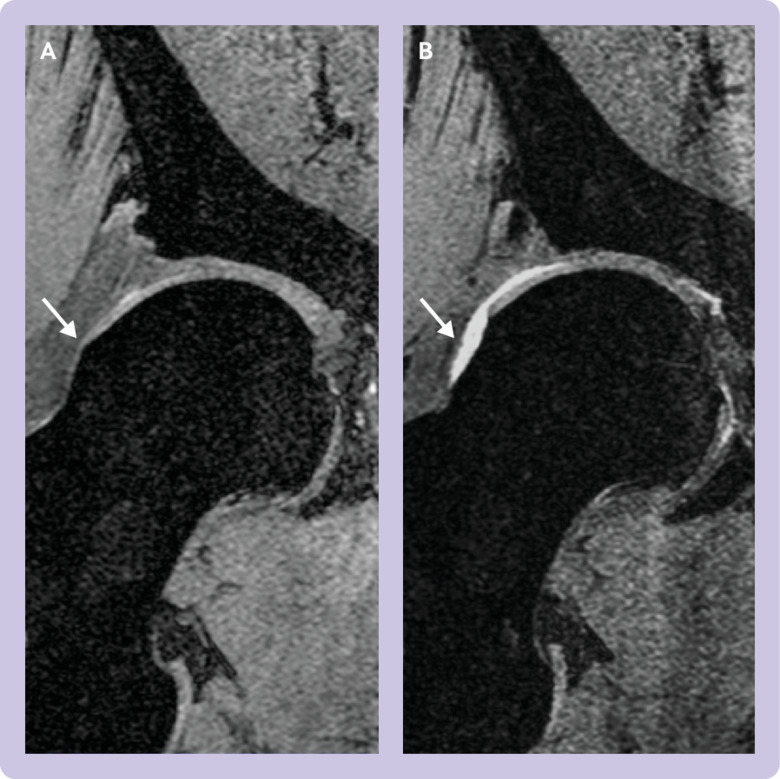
Right hip coronal magnetic resonance image of trial participant randomised to surgery: (A) Baseline image showing cam morphology (arrow). (B) Six months after hip arthroscopy with restoration of the normal concavity at the femoral head-neck junction by burring away the cam lesion (arrow). This procedure prevents abutment of the femoral head-neck junction against the acetabular rim during a functional range of movement
